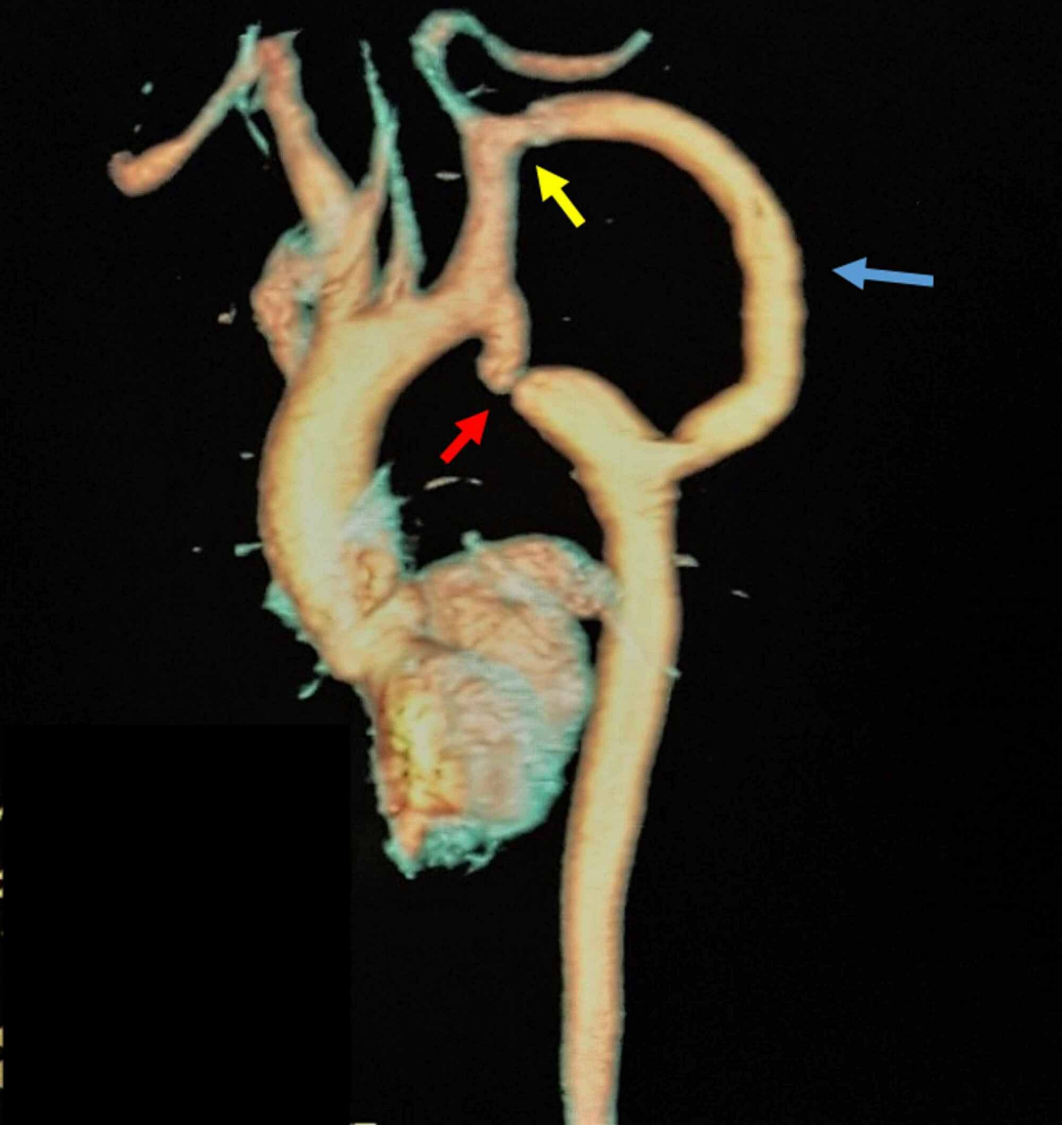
Computed tomography scan of the aorta revealing severe aortic coarctation (red arrow), extra-anatomic bypass graft from the left subclavian artery to the descending aorta (blue arrow), and stenosis at the proximal anastomosis site (yellow arrow)
